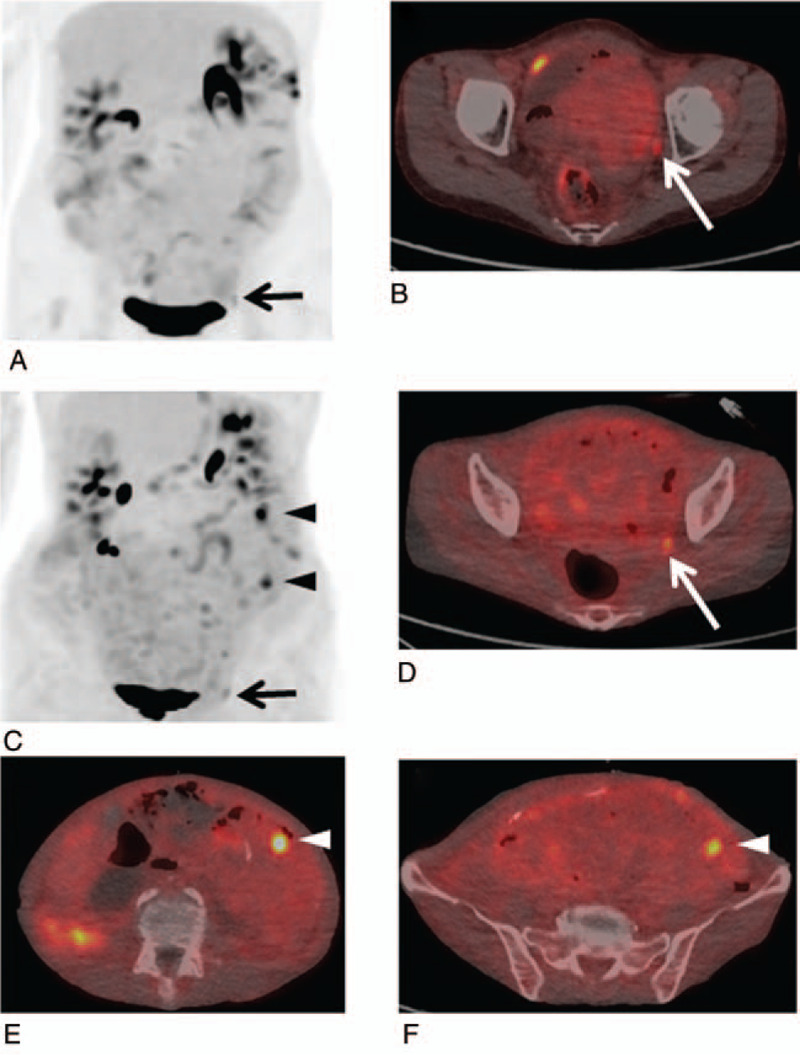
A 61-year-old woman with postoperative recurrence gastric cancer with peritoneal metastasis (stage IV) received nivolumab. Baseline ^18^F-FDG-PET/CT [MIP (A) and fused transaxial (B) images] shows abnormal ^18^F-FDG uptake in the peritoneal metastasis in the left peritoneal cavity (A and B: arrows). The follow-up ^18^F-FDG-PET/CT after 9 courses of nivolumab therapy [MIP (C) and fused transaxial (D–F) images] show the progression of known peritoneal metastasis (C and D: arrows) with new hypermetabolic peritoneal metastases (C, D, and F: arrowheads). The increase of the sum of the SUVmax and SULpeaks were 178.1% (2.65–7.37) and 255.0% (1.42–5.04), respectively. The status was PMD according to EORTC or PERCIST. The patient exhibited progressive disease at 4 mo and died 5 mo after the initiation of nivolumab.
